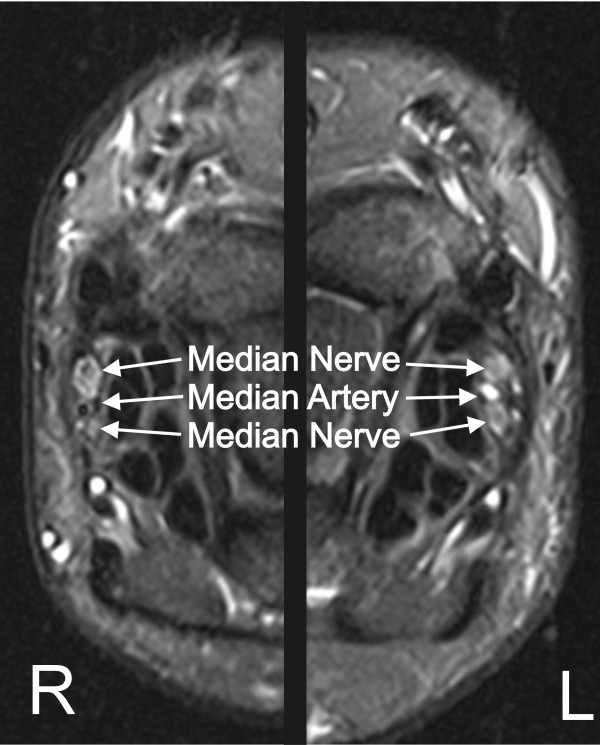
MR image of bilateral persistent median artery. Axial STIR-w images of the right and left wrists of a subject with bilateral CTS symptoms showing a persistent median artery within the bifurcated median nerve.
